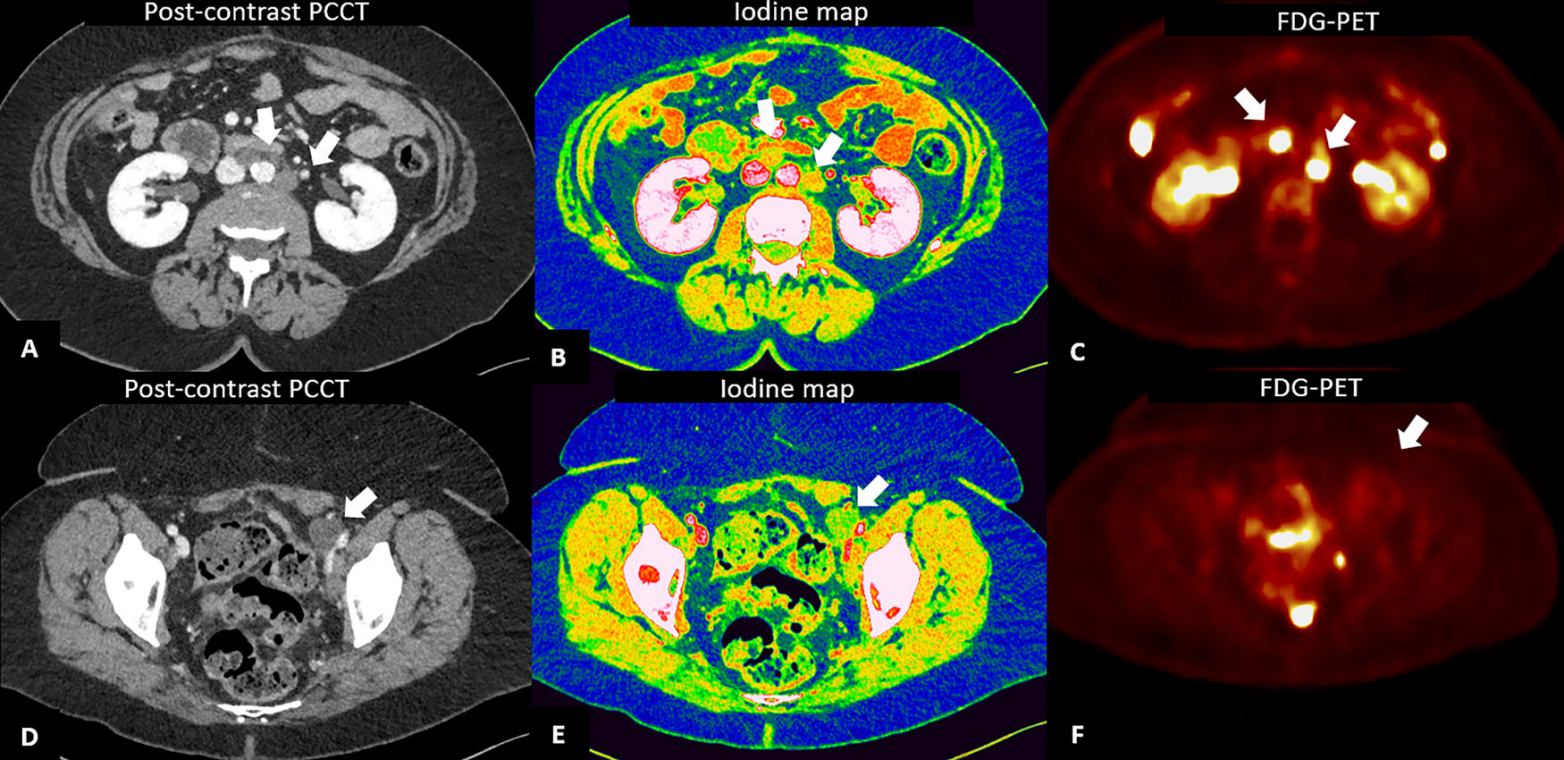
Lymph node metastases detection with PCCT. A 65-year-old woman with a history of oophorectomy for ovarian cancer 3 years prior, followed by immunotherapy for peritoneal carcinosis 2 years later, undergoing CT due to rising tumor markers and suspicion of cancer relapse. Angiographic axial scan (A–D) shows multiple rounded nodes along the abdominal aorta and iliac arteries, with a maximum short axis of 15 mm. The periaortic nodes showed iodine uptake on iodine map (B), suggestive of pathological involvement. In contrast, the left iliac node (arrow in D) shows no iodine uptake on the iodine map (E), primarily suggestive of lymphocele post-lymphoadenectomy. These results were confirmed at FDG-PET, which showed FDG uptake in the para-aortic nodes (C), and no uptake of the para-iliac node (F)
